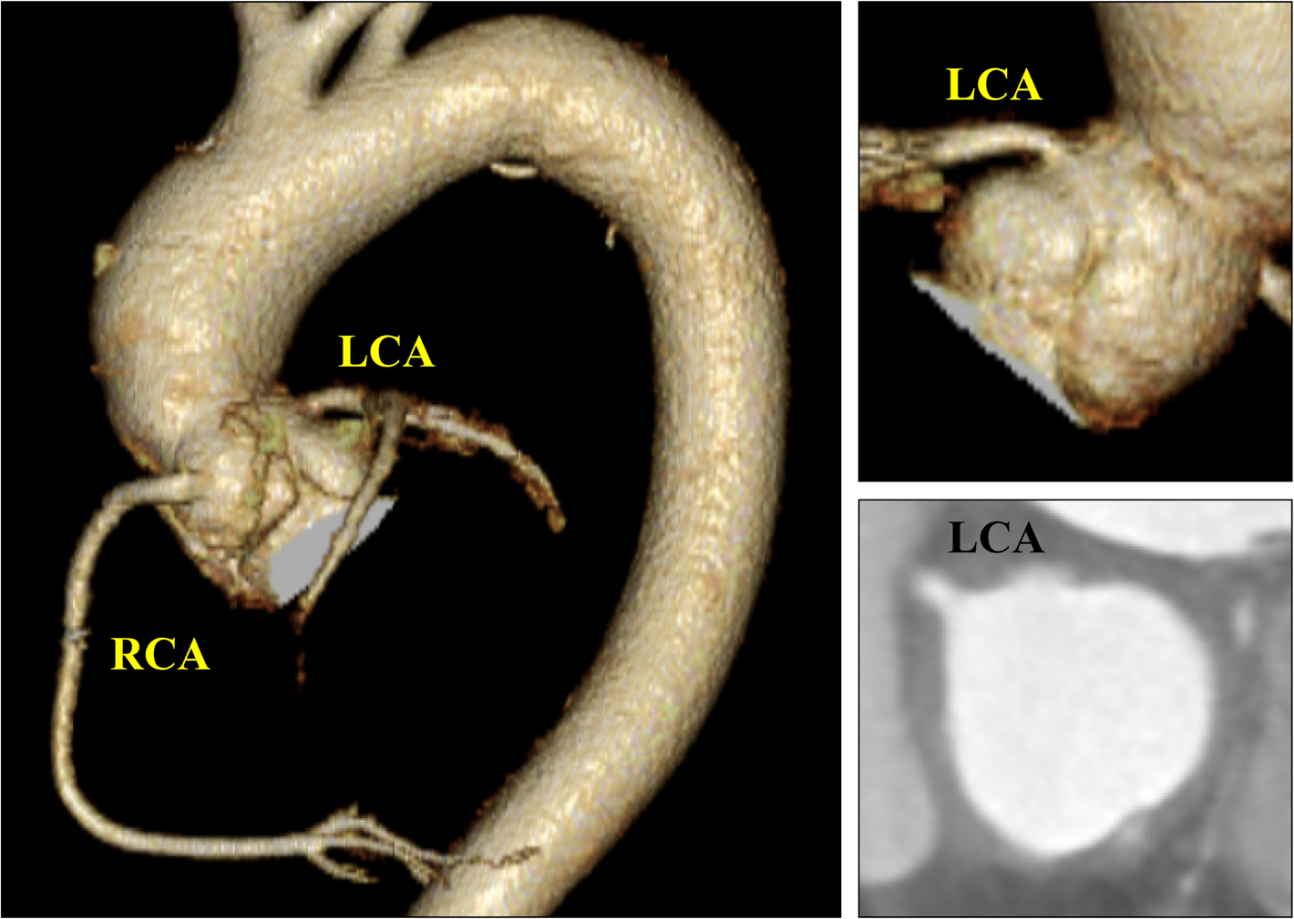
Computed tomography taken 2 years after the operation. There was no distortion or stenosis associated with the covered graft at the left main coronary artery trunk. RCA: right coronary artery; LCA: left coronary artery
